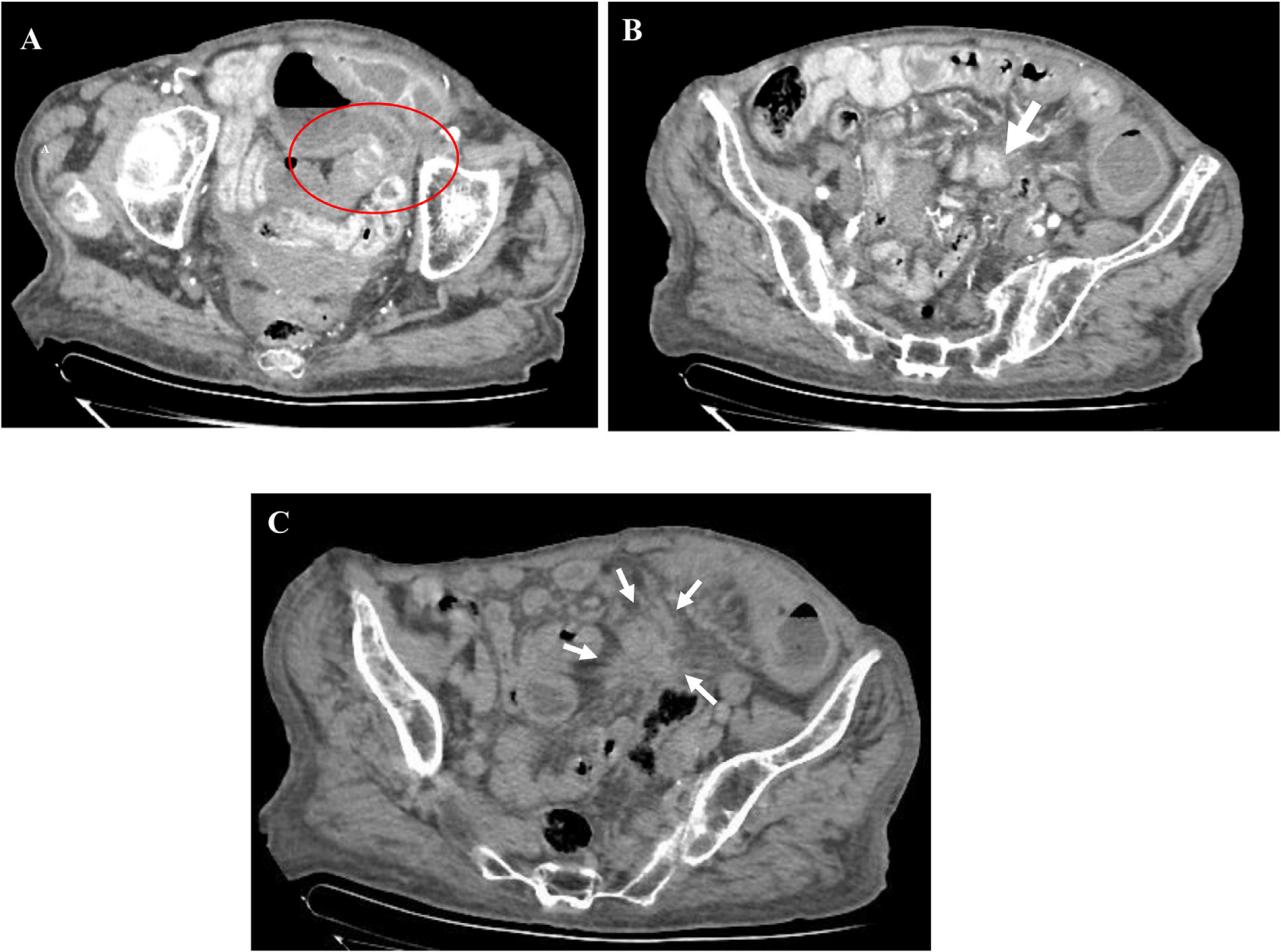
Axial enhanced abdominal CT scan: A, B: arterial phase, C: Portal phase, objectiving: (A) Endoluminal polypoid hypervascular lesion (red circle). (B) Hypervascular mesenteric retractil mass (arrow). (C) Spiculations with stranding bands around the mesenteric mass (arrows)
